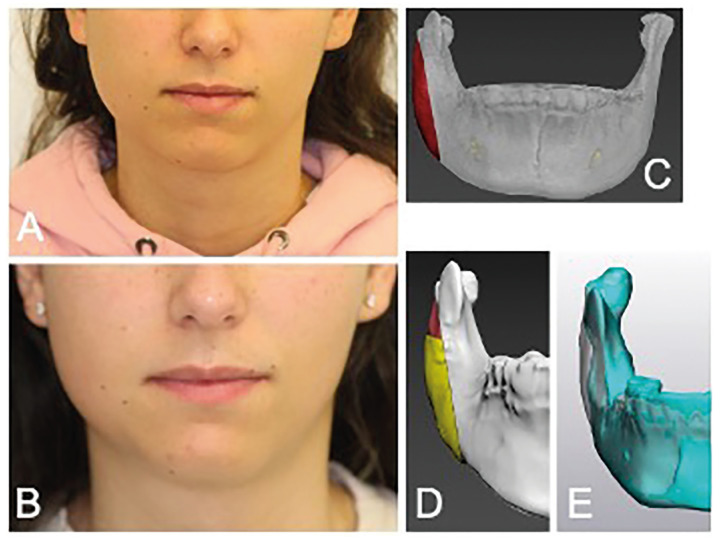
A 19-year-old patient with DSO/TP of the right mandible. (A + B) Pre- and post-operative pictures with a decrease in asymmetry on the right side. (C + D) Three-dimensional reconstruction (3D) of CBCT-scan on which the osseous swelling can be seen, with a planning for remodelling surgery. In red a mirrored model to determine the asymmetry and to determine the resection osteotomy, and in yellow the matching patient-specific surgical guide. (E) 3D reconstruction of the pre- (transparent) and postoperative (blue) situation, showing a more symmetrical appearance.
